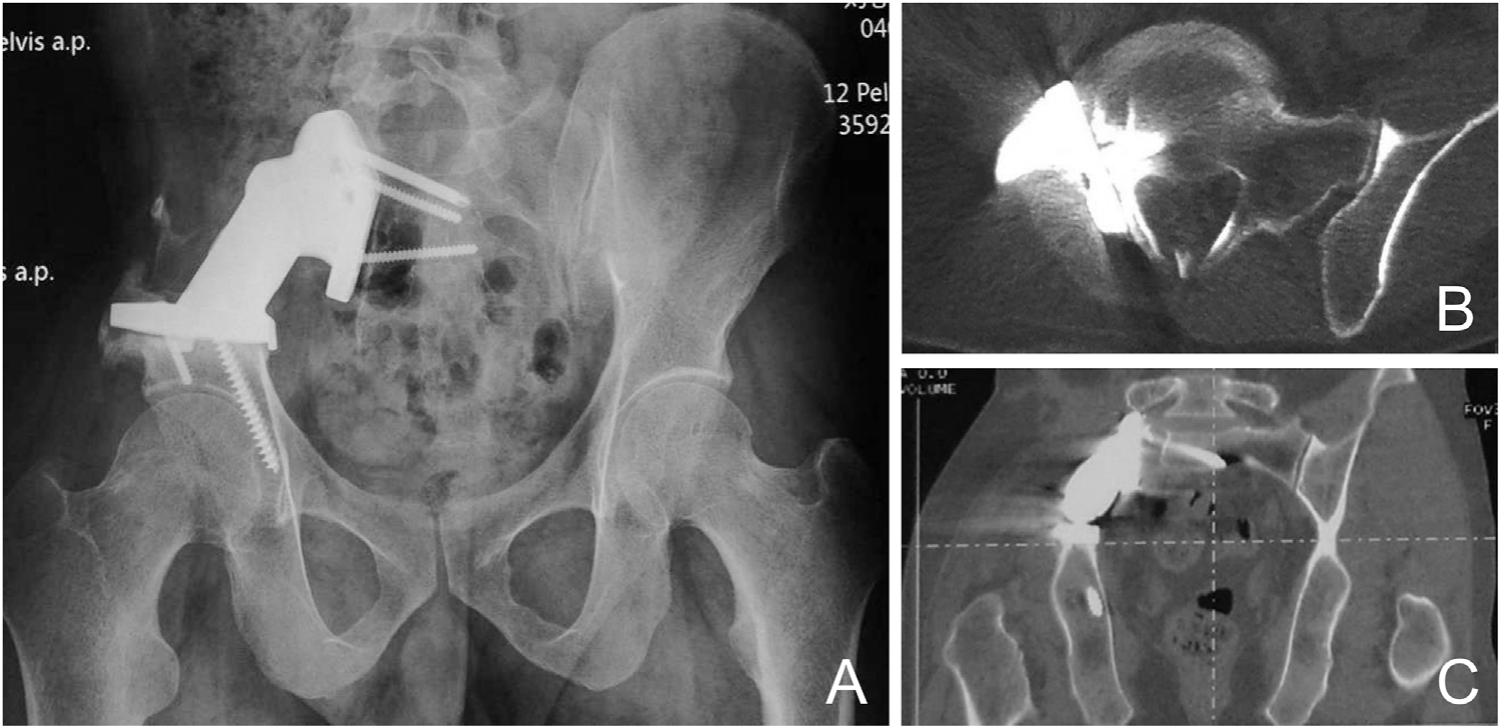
Prosthetic Osseointegration (A) X-rays show a well positioned prosthesis with no use of loose displacement or fracture. CT shows good osseointegration of the implant with the adjacent bone in the sagittal (B) and coronal planes (C).
